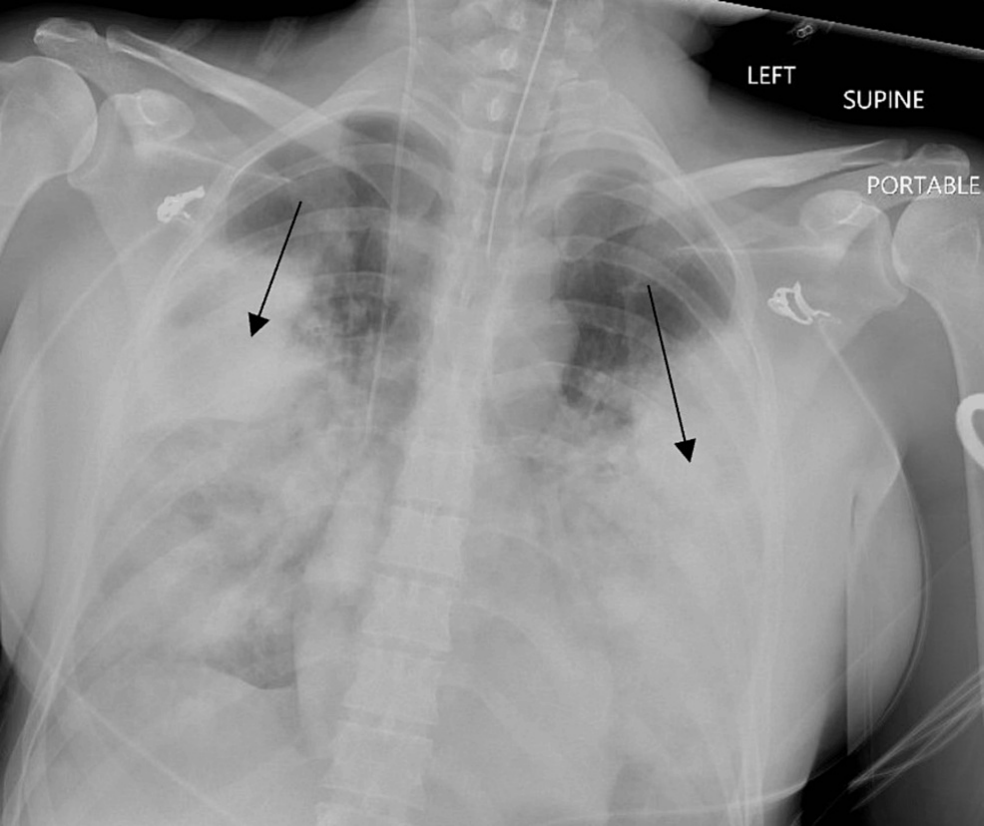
Chest X-ray obtained in the emergency department demonstrates dense infiltrates within the mid-lung fields bilaterally. A right internal jugular central line terminates in the mid-superior vena cava. The endotracheal tube remains in place. The heart is within normal limits for size. No effusion or pneumothorax is seen.
